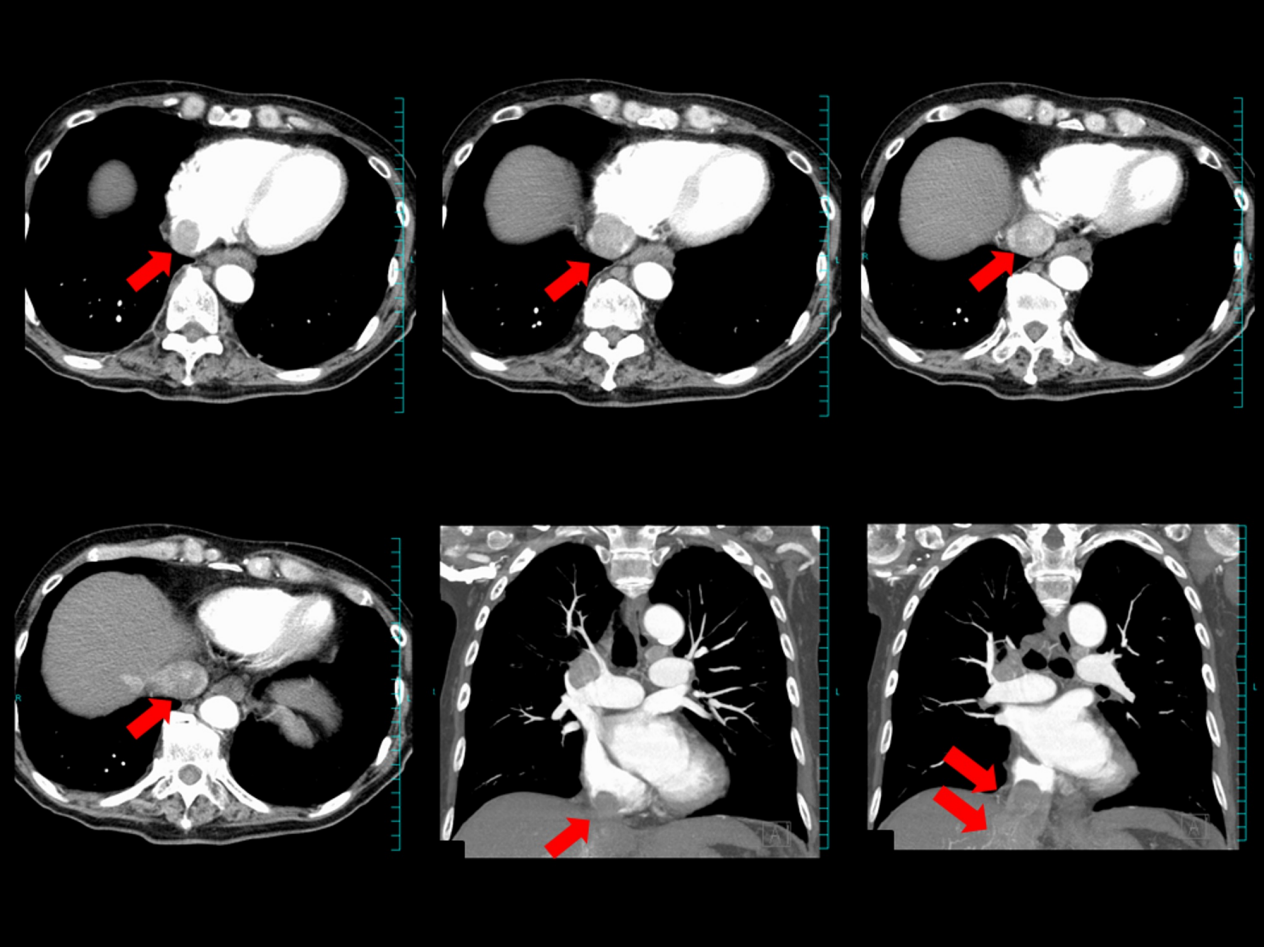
Contrast CT scan at diagnosis. Massive IVC tumor (red arrow) is protruding into the right atrium. IVC, inferior vena cava
